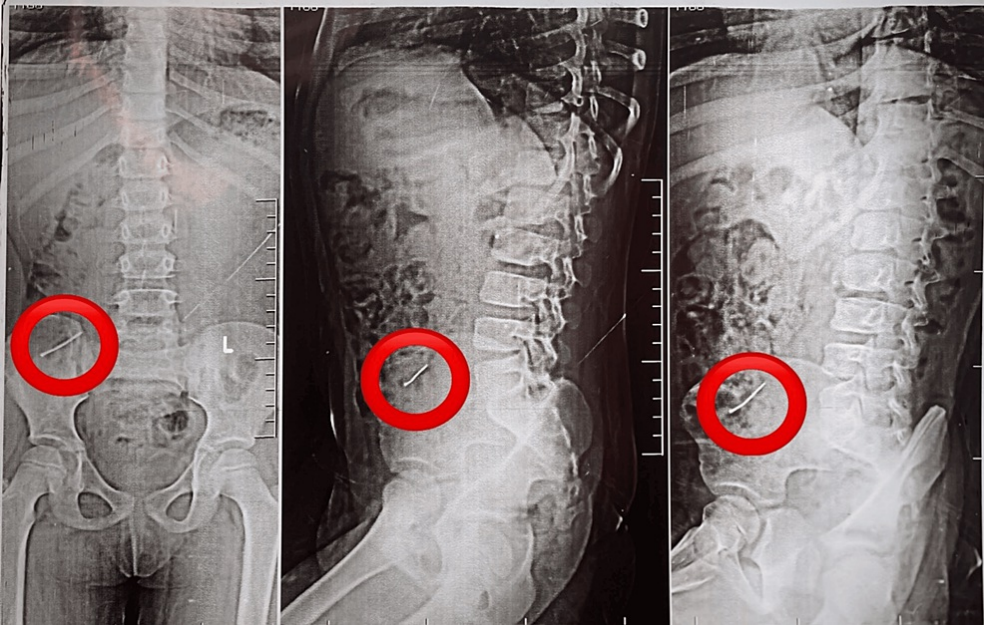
A plain film X-ray shows a radiopaque foreign body in the right iliac fossa
